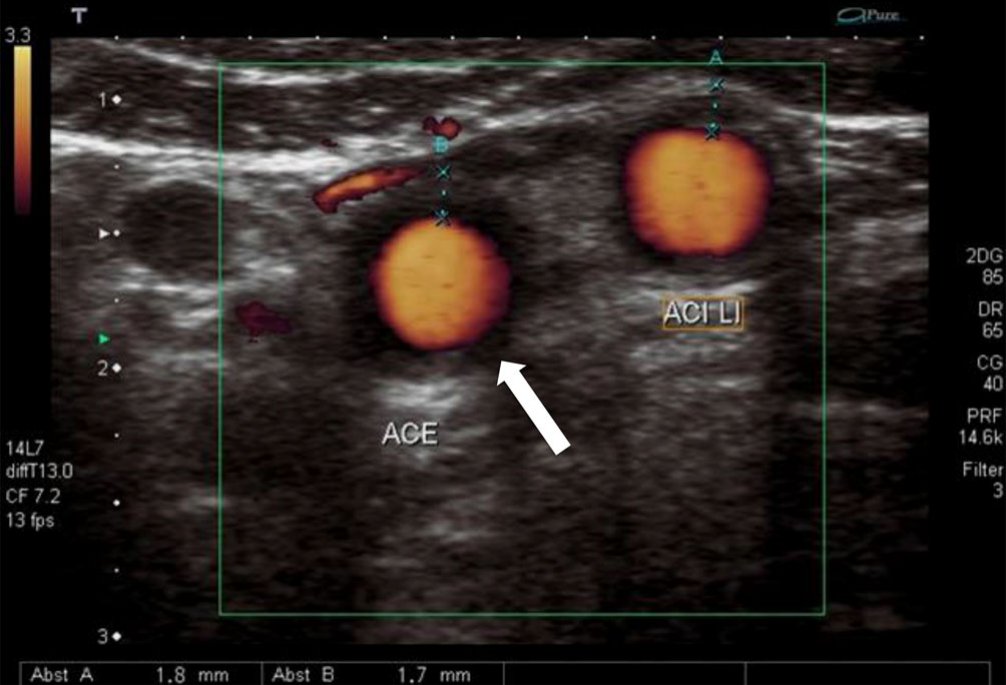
Giant cell arteritis with involvement of the extracranial carotid artery and typical halo (*arrow*) on color duplex sonography (courtesy of Dr. G. Herzog)
